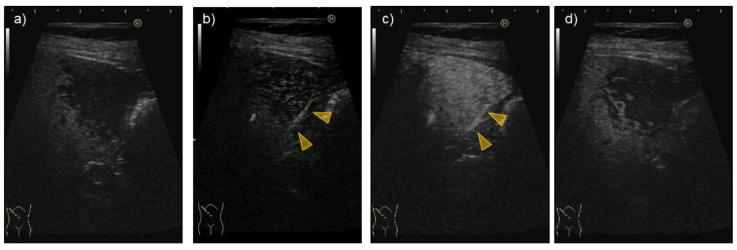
The tumor lesion at segment S5 in the liver was hypo-echoic on B-mode ultrasonography (a), through which a penetrating vessel was detected (b, arrowheads), which was enhanced in the arterial phase (c, arrowheads). The vessel was evident in the Kupffer phase (d) on enhanced ultrasonography using Sonazoid.
